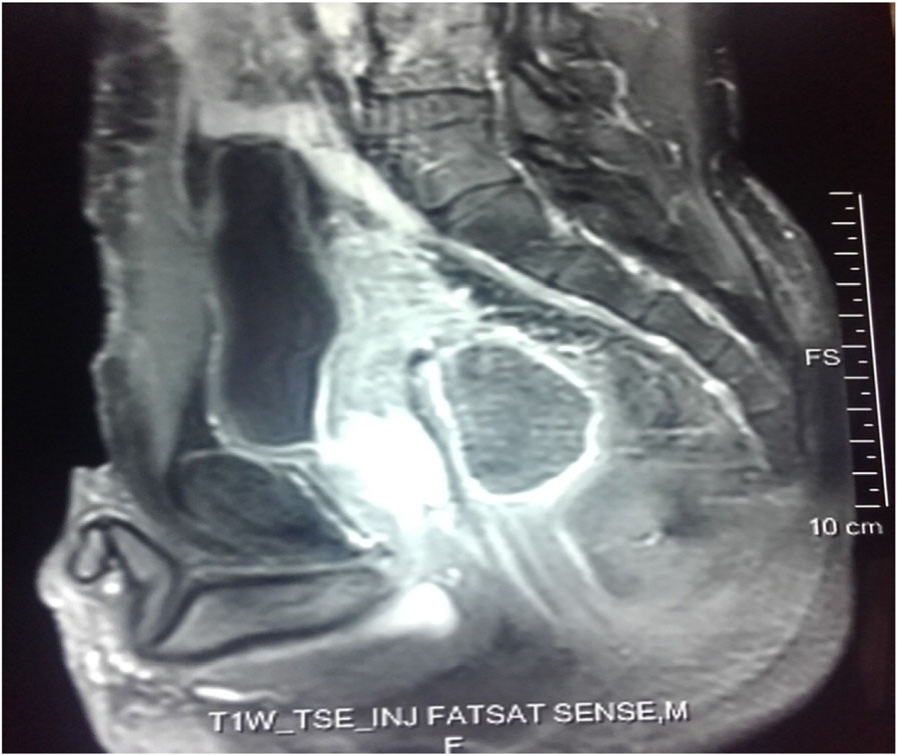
Image showing sagittal view of a T2-weighted magnetic resonance image of the pelvis, which showed no evidence of recurrence
